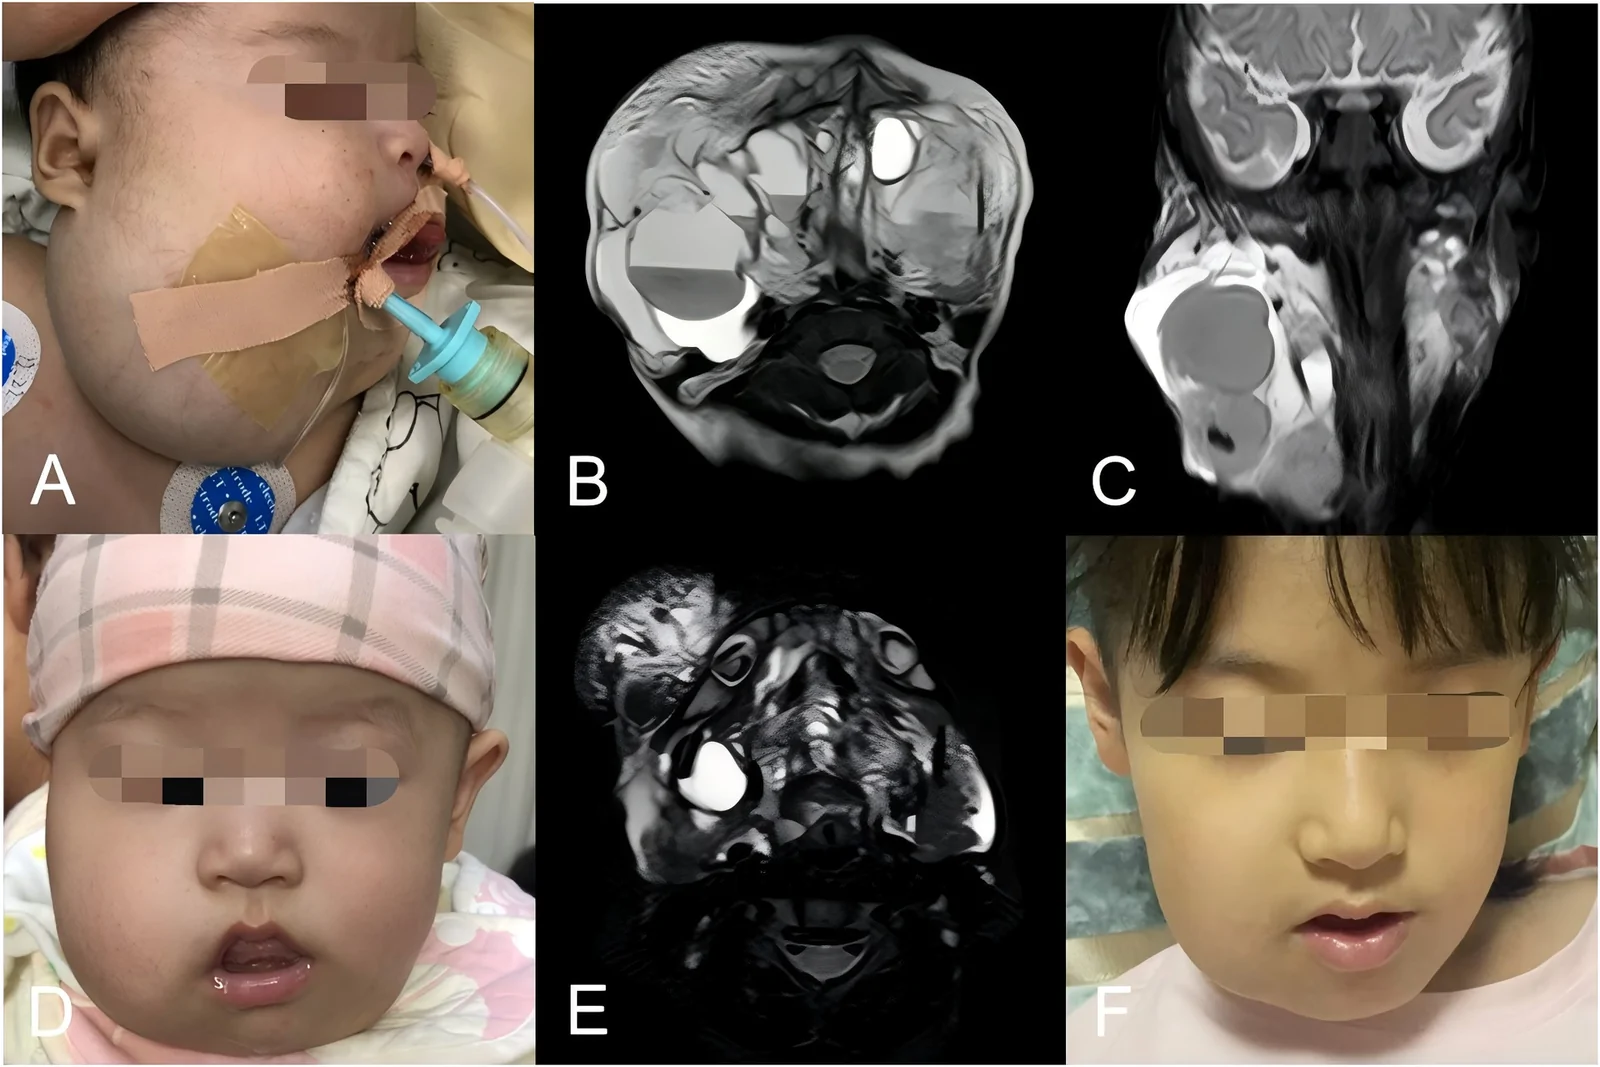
(A) A preoperative photograph demonstrated diffuse swelling of the maxillofacial and cervical regions, with non - pulsatile oral haemorrhage. (B–C) Preoperative axial and coronal MRI sequences demonstrated extensive abnormal signal intensities in the subcutaneous tissue, tongue, sublingual, submental, and cervical regions. The lesion presented as multiloculated cysts of varying sizes, with focal fluid - fluid levels. This led to a marked narrowing of the airway, with a significantly reduced cross - sectional area, and a leftward (contralateral) deviation of the airway from its normal tubular. (D–E) 3-month follow-up postmanagement photograph showed a significant reduction of the size of the massive maxillofacial and cervical swelling. MRI revealed remarkable improvement of the orofacial lymphatic malformation compared with before. There was no evidence of recurrence or hemorrhage. (F) At the 7-year follow-up: Clinical photograph showed persistent clinical stable.
